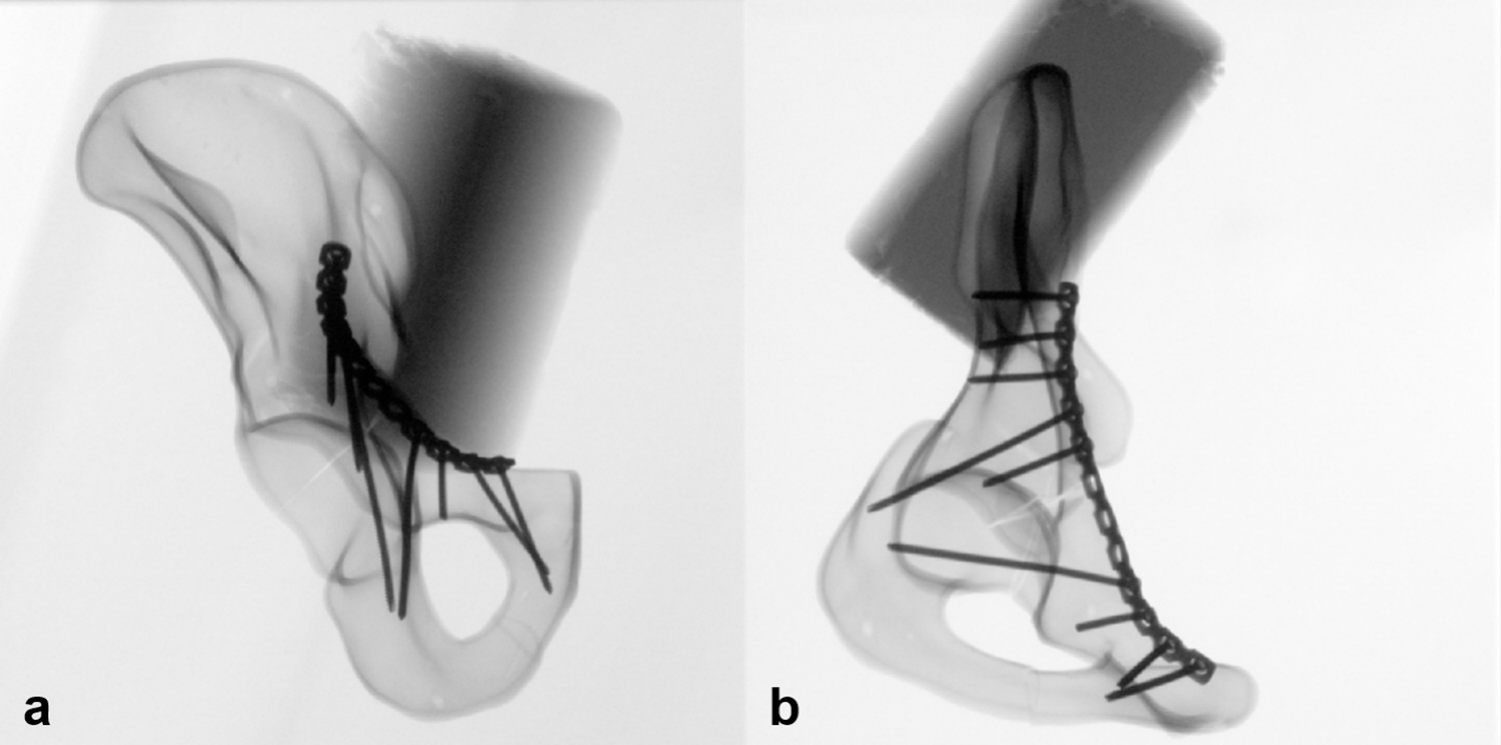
X-ray imaging of the pelvis model with plate osteosynthesis. a shows the a.p.- view and b the obturator- view of the hemi-pelvis.
